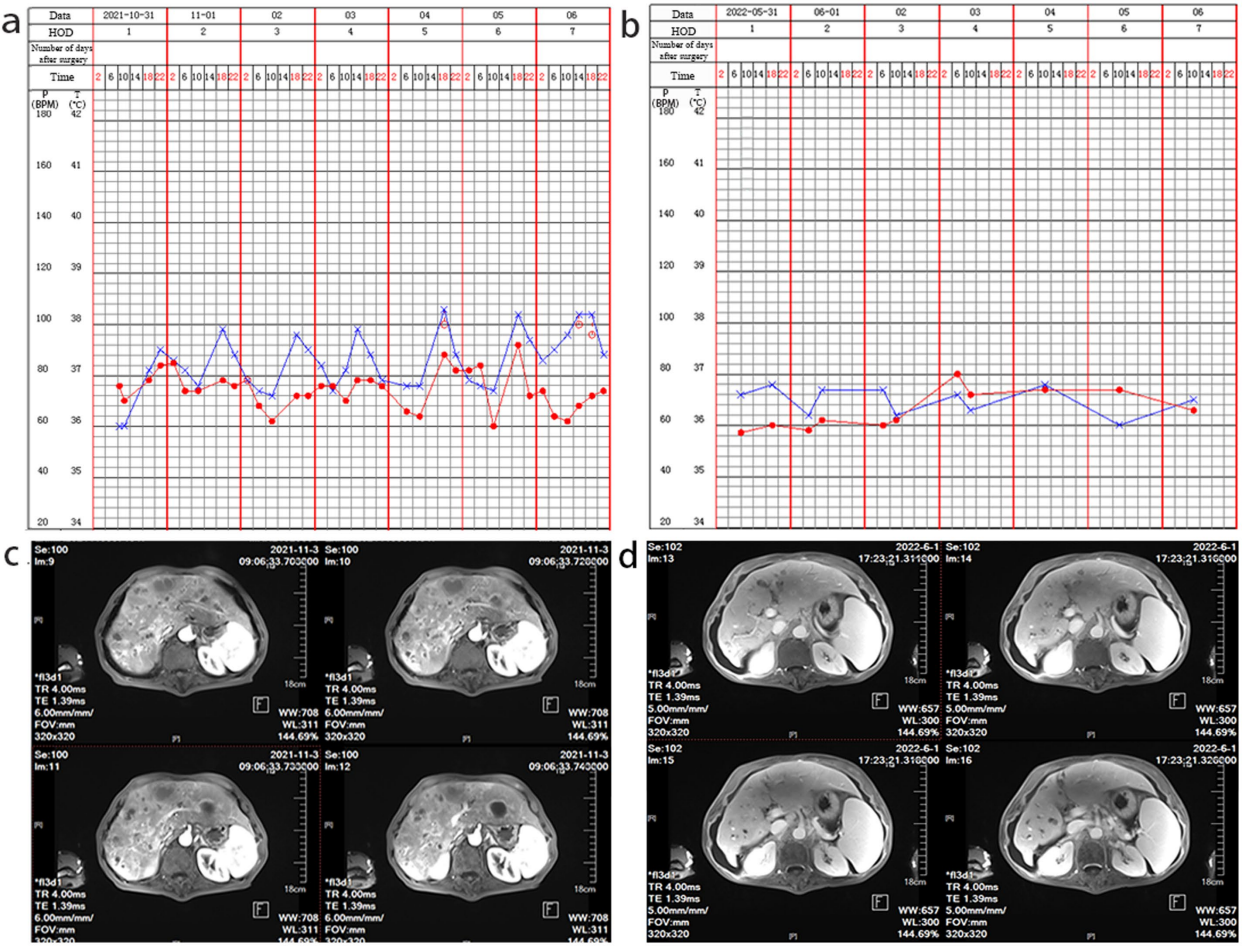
Nursing record sheet and liver MRI examination. (a) At the time of admission, a low fever was observed. (b) A follow-up after treatment indicated that the admission examination reveals a normal body temperature. (c) The initial liver MRI examination shows hepatic space-occupying lesions; (d) After treatment, a follow-up liver MRI examination shows improvement in liver abscess.
